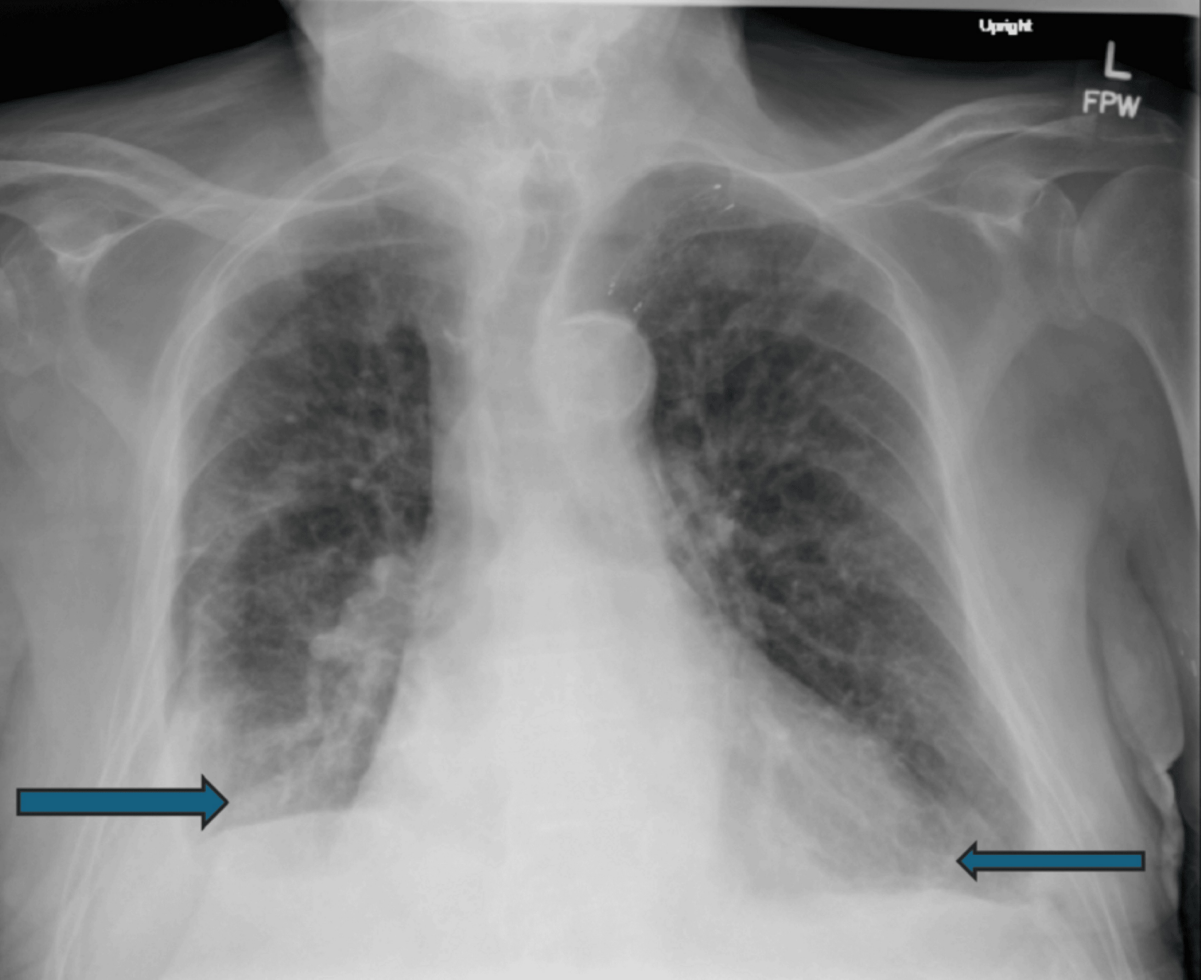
Chest radiograph in early July 2025, two days after bilateral therapeutic thoracentesis
